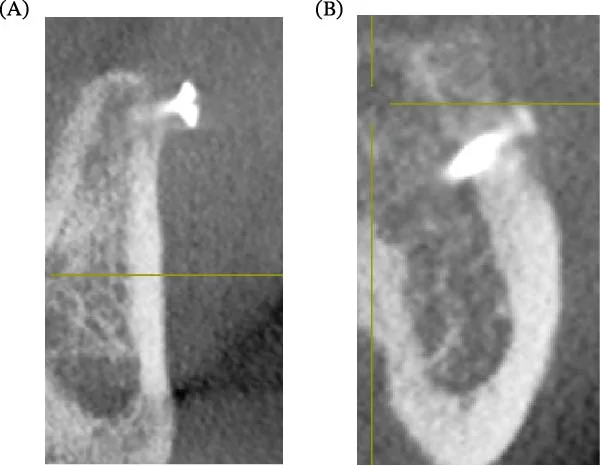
Sectional CBCT scan in coronal section showing the head of the osteosynthesis screw used as reference. (A) Significant bone resorption represented by bone shadow between the internal side of screw head and the superior surface of the bone graft. (B) Stable bone with zero bone resorption around the head of the screw.
